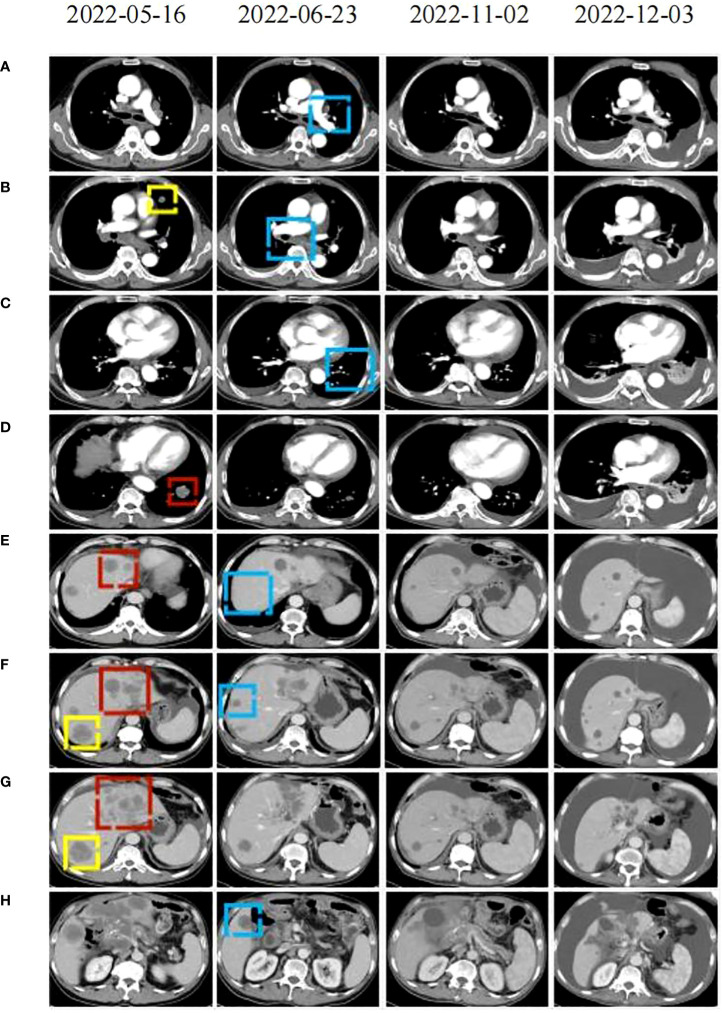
CT scans were conducted before (2022-05-16), during (2022-22-02), and after (2122-12-03, 2021-05-12) the PRaG3.0 therapy. The baseline inspection was performed on 2022-05-16 (A–H): the red area indicates the first cycle of radiation, the yellow area represents the second cycle of radiation. The radiographic changes after two cycles of treatment were observed on 2022-06-23 (A–H): the blue area indicating tumor regression in the unirradiated area. During maintenance treatment. On 2022-11-02 (A–H): changes in the patient’s lesion were noted. On 2022-12-03 (A–H), the tumor lesion progressed and thoracic and abdominal effusion developed.
